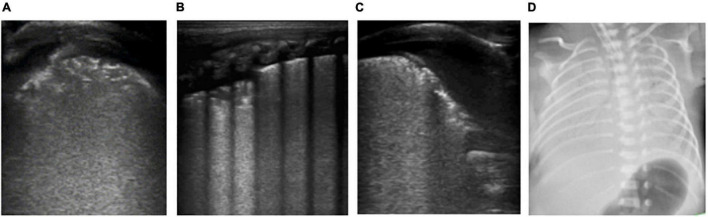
The patient is a twin neonate born at 32 weeks of gestational age by Cesarean section due to “fetal distress” with clear amniotic fluid and the Apgar score of 1, 5, and 10 min of birth were 5, 7, and 7 points. After delivery, the neonate had cyanosis, labored breathing, and severe pulmonary hypertension, and high-frequency ventilation was performed. It was diagnosed as neonatal respiratory distress syndrome (RDS). (D) Chest X-ray: White lung changes. (A–C) LUS: Abnormal pleural line, the disappearance of A-lines, white lung change, snowflake sign, such as air bronchogram visible below pleura, which conforms to the ultrasonic characteristics of severe RDS.
